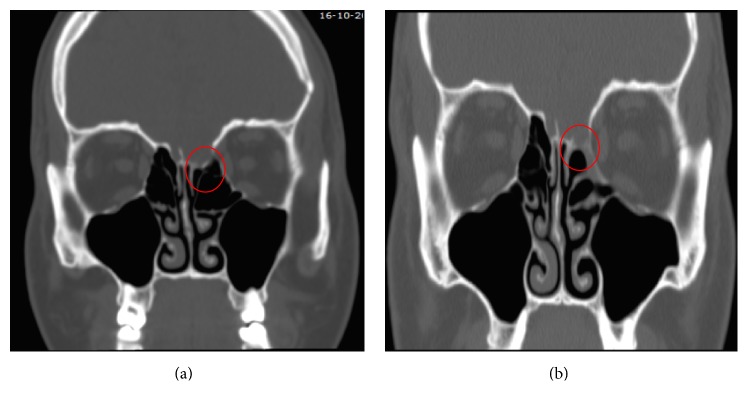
Case 2. (a) Preoperative CT scan showing a CSF leak in the left cribriform plate. (b) Seven-month postoperative CT showing a less dense bony closure of the surgical defect.
